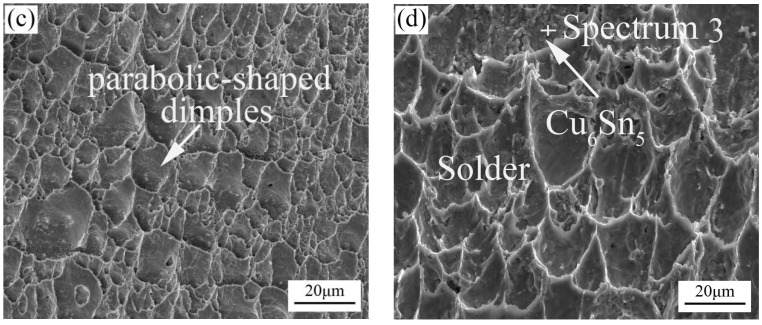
Top views of the shear fracture surfaces for the solder joints at a 400-ms ultrasonic bonding time for different aging times: (a) 24 h; (b) 120 h; (c) 240 h and (d) 360 h.
